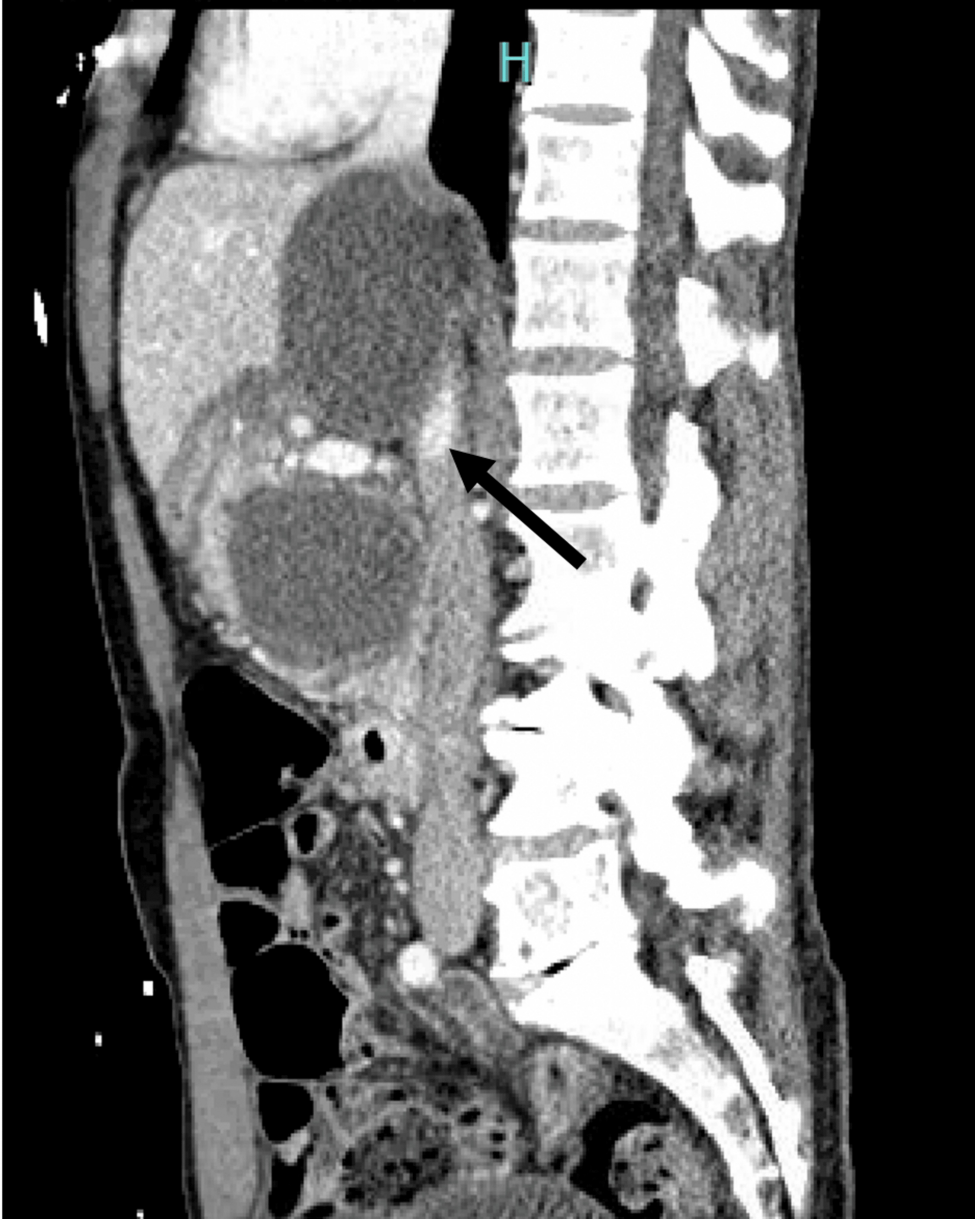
Sagittal CT abdomen and pelvis showing severe superior mesenteric vein compression (arrow) CT, computed tomography
